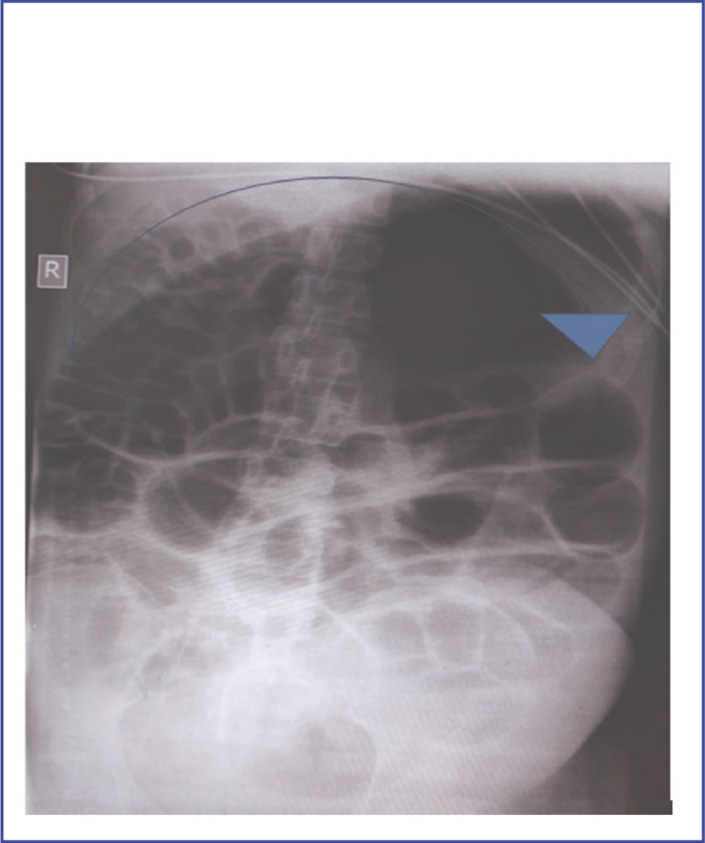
Plain abdomen radiography of the patient showing a dilatation of proximal part of the colon up to approximately the splenic flexure (Blue arrow).
